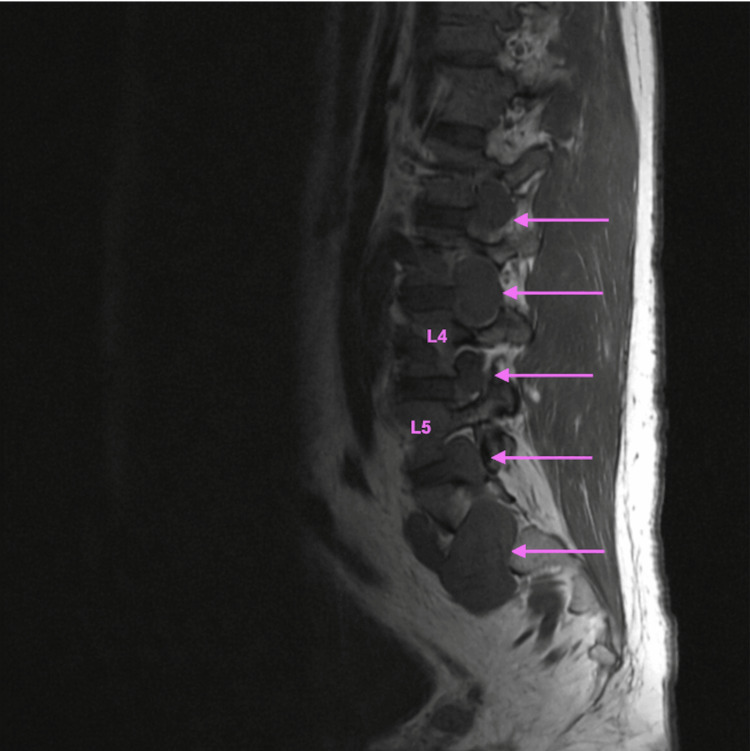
Parasagittal T1-weighted MRI MRI demonstrating marked hypertrophy of non-enhancing lumbosacral nerve roots extending through the neural foramina (purple arrows).
